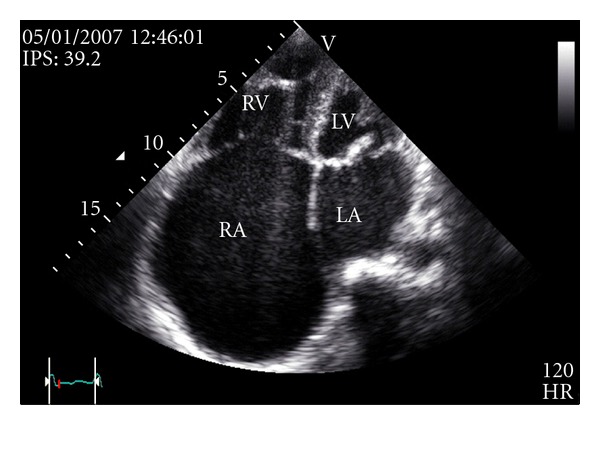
Transthoracic echocardiography, apical four chambers view. Massive enlargement of the right atrium. The right atrial area was 80.6 cm² (53.7 cm²/m²), and the calculated right atrial volume was 621 mL (414 mL/m²). LA: left atrium. LV: left ventricle. RA: right atrium. RV: right ventricle.
